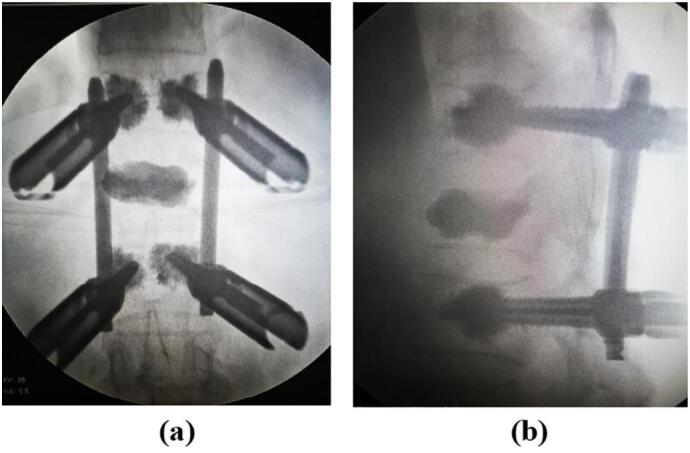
Intraoperative positive and lateral X-ray.
